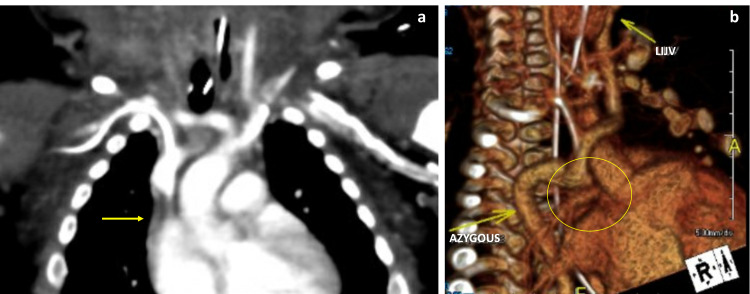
(a) CT angiography showing near-complete occlusion of SVC. (b) CT angiography showing near-complete occlusion of SVC and right internal jugular vein with a large dilated azygous vein and left internal jugular vein (LIJV).
